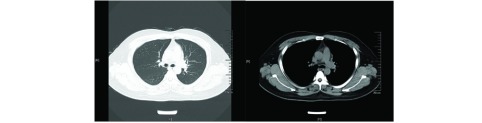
胸部CT（2017-02-17）：左肺门软组织影，大小约3.2 cm×2.4 cm，分叶状，密度尚均匀，周围可见肿大淋巴结影。 Chest CT scan (2017-02-17): A lobulated soft tissue giant tumor located in the left pulmonary hilum with the size of about 3.2 cm×2.4 cm showed homogeneous density; lymphadenopathy around it can be seen.
